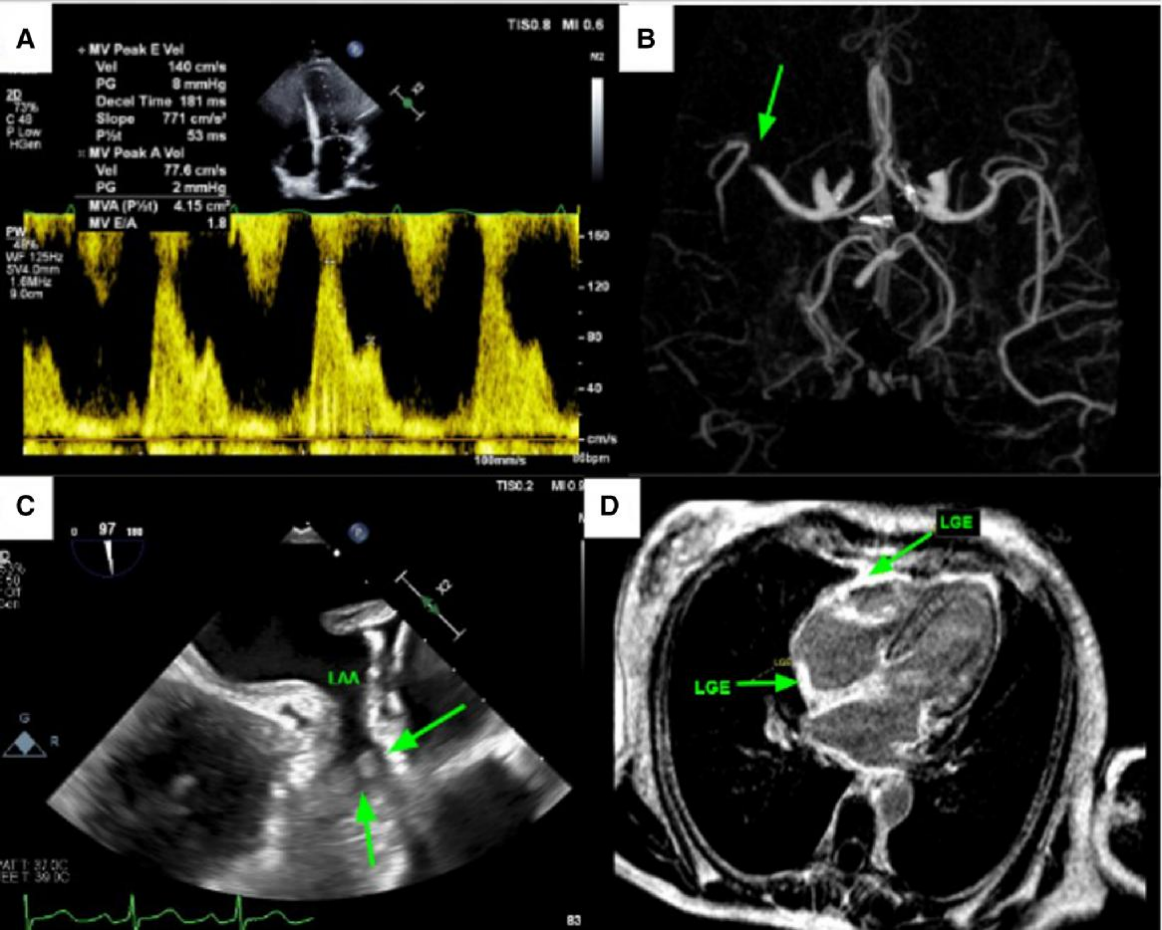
(*A*) Restrictive mitral valve inflow Doppler (transthoracic echocardiogram). (*B*) Computed tomography perfusion brain reveals right middle cerebral artery occlusion indicated by an arrow. (*C*) Transoesophageal echocardiogram reveals left atrial appendage thrombus indicated by arrows. (*D*) Cardiac magnetic resonance imaging reveals diffuse infiltrate and fibrosis indicating cardiac amyloidosis.
